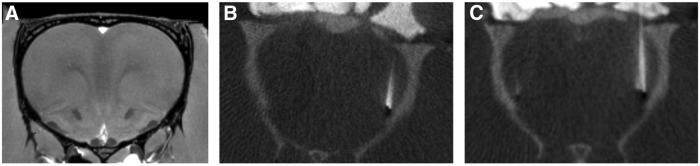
Example of registration between individual CT scans and the MRH atlas. *A*, Coronal slice of MRH atlas. *B*, Corresponding slice in unregistered CT. *C*, Corresponding slice in registered CT.
